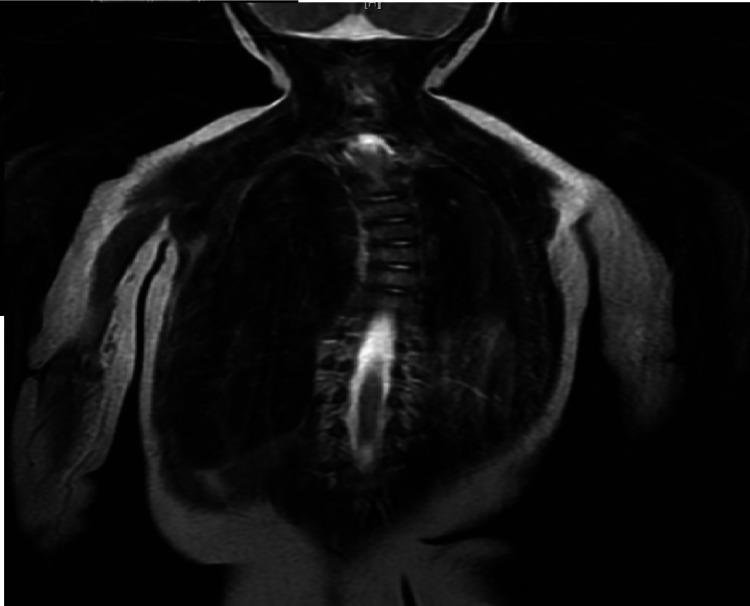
MRI showing levocurvature and dextrocurvature of the thoracic and lumbar spine, respectively. Note incidental finding of hydromyelia extending from T10-11 to T12-L1.
